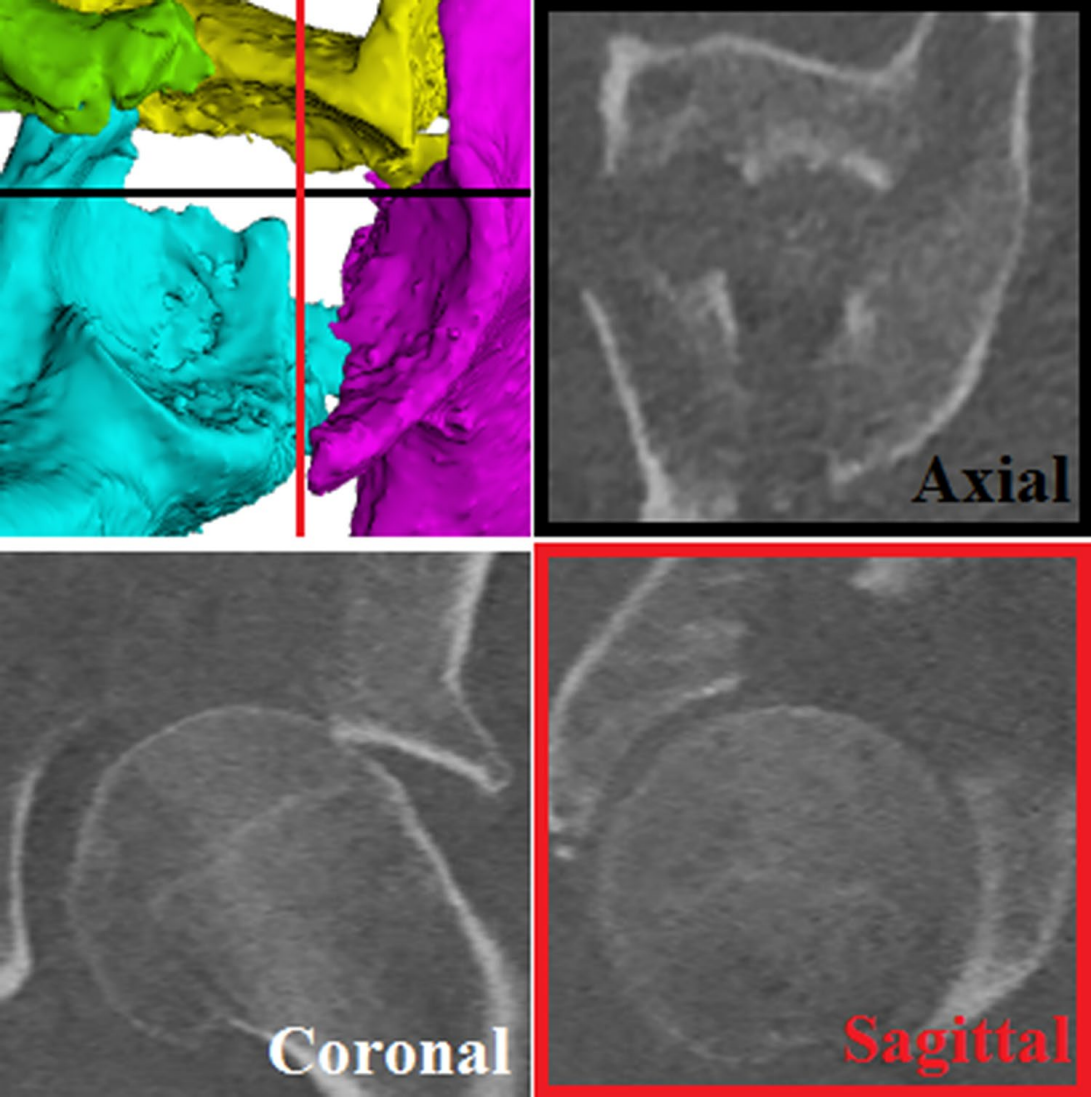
A gap and step-off can be seen on a 3D view of the acetabulum (top left). The black line represents the axial plane and the red line represents the sagittal plane. Multiple fracture lines, with gaps and step-offs, can be seen on the CT slices.
